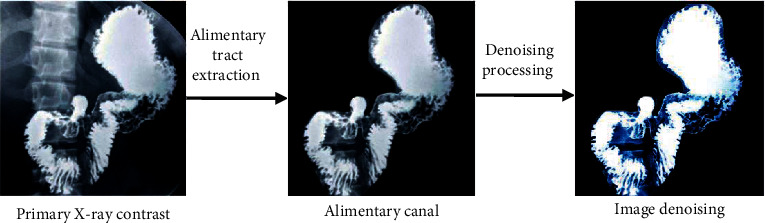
The processing process of gastrointestinal X-ray imaging using intelligent algorithm.
